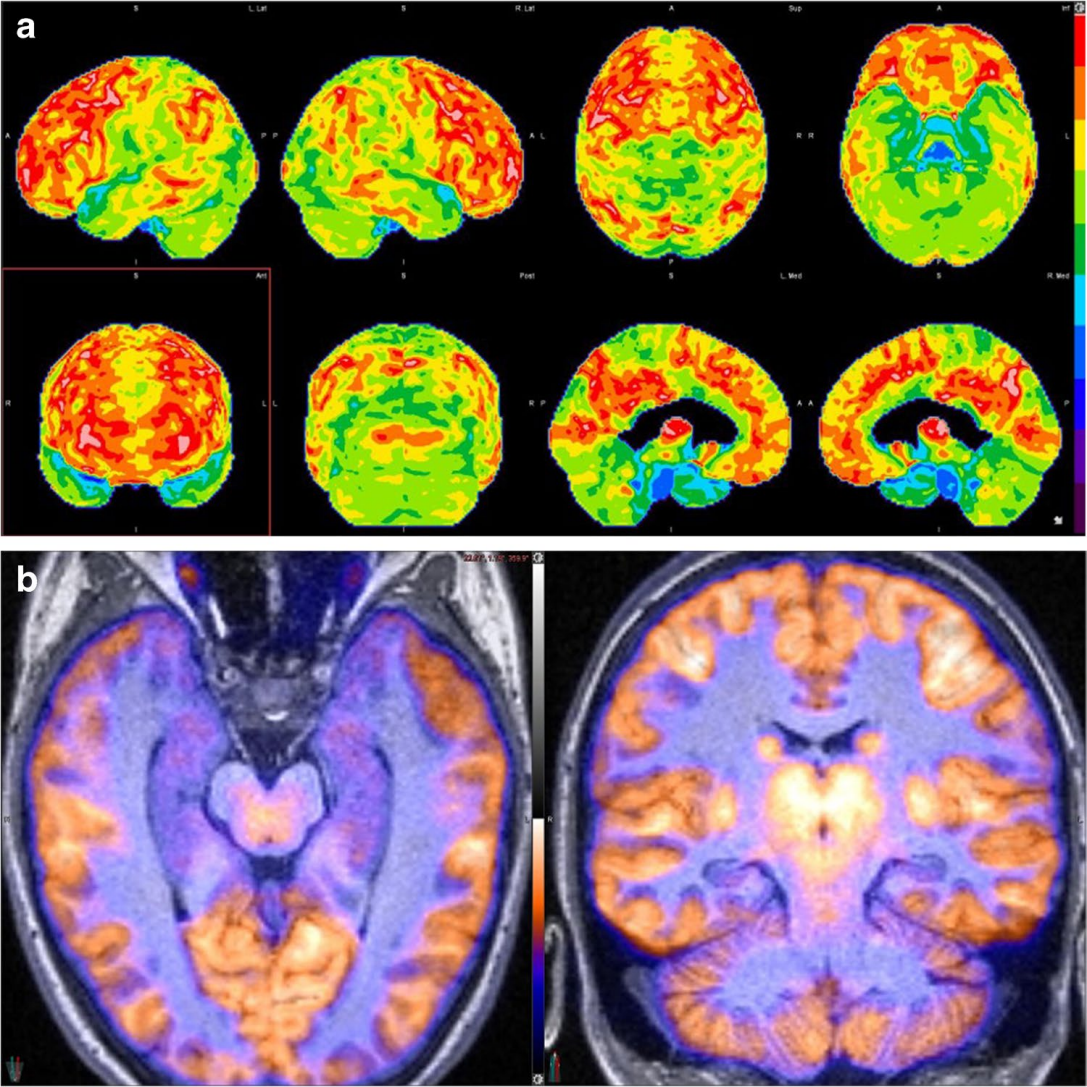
a 3D-SSP analysis of ^18^FDG PET normalized to the whole brain shows mild-to-moderate FDG hypometabolism in the bilateral anterior and medial temporal lobes, superior parietal lobes, lateral occipital lobes, anterior cingulate cortices, and bilateral cerebellar hemispheres. b
